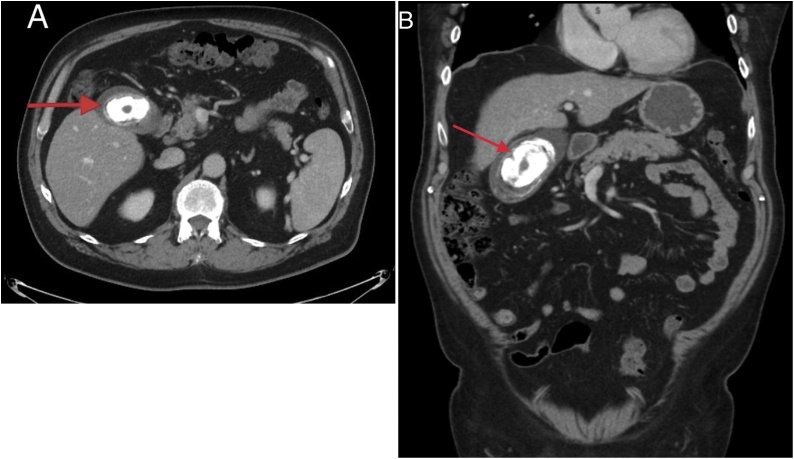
Axial (a) and coronal (b) abdominal/pelvic CT images showing large calcified gallstone in gallbladder.
